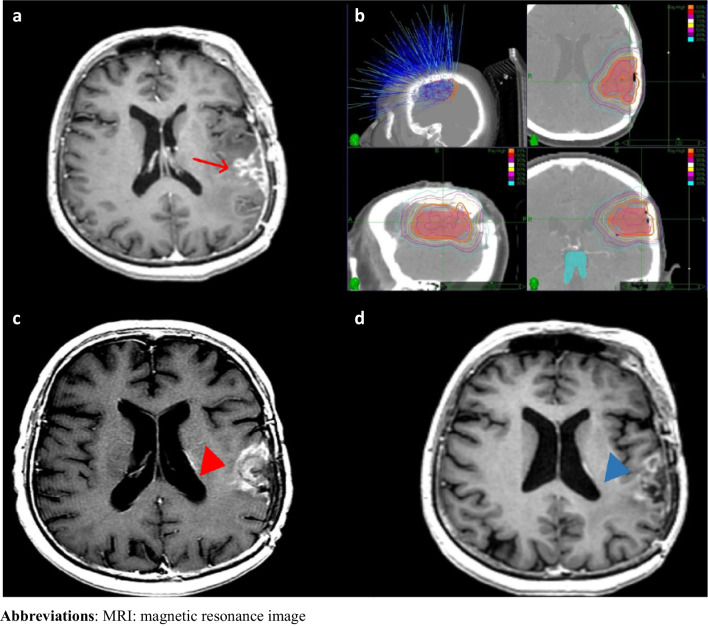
This figure depicts the treatment and follow-up images of a patient diagnosed with recurrent glioblastoma a. T1 post-contrast (T1c +) sequence of the MRI for reirradiation planning (red arrow: contrast enhanced recurrent lesion at left temporoparietal lobe) b. Treatment plan (dose is prescribed to the 83% isodose line, orange isodose line represents 83% isodose) c. At 6 months, MRI showed an increase in contrast-enhancing lesion (red arrowhead) on axial T1C + sequence, pseudoprogression and progression were included in the differential diagnosis d. At 8 months after reirradiation, MRI revealed a decrease in contrast-enhancing lesion (blue arrowhead), confirming the presence of pseudoprogression. Abbreviations: MRI: magnetic resonance image
